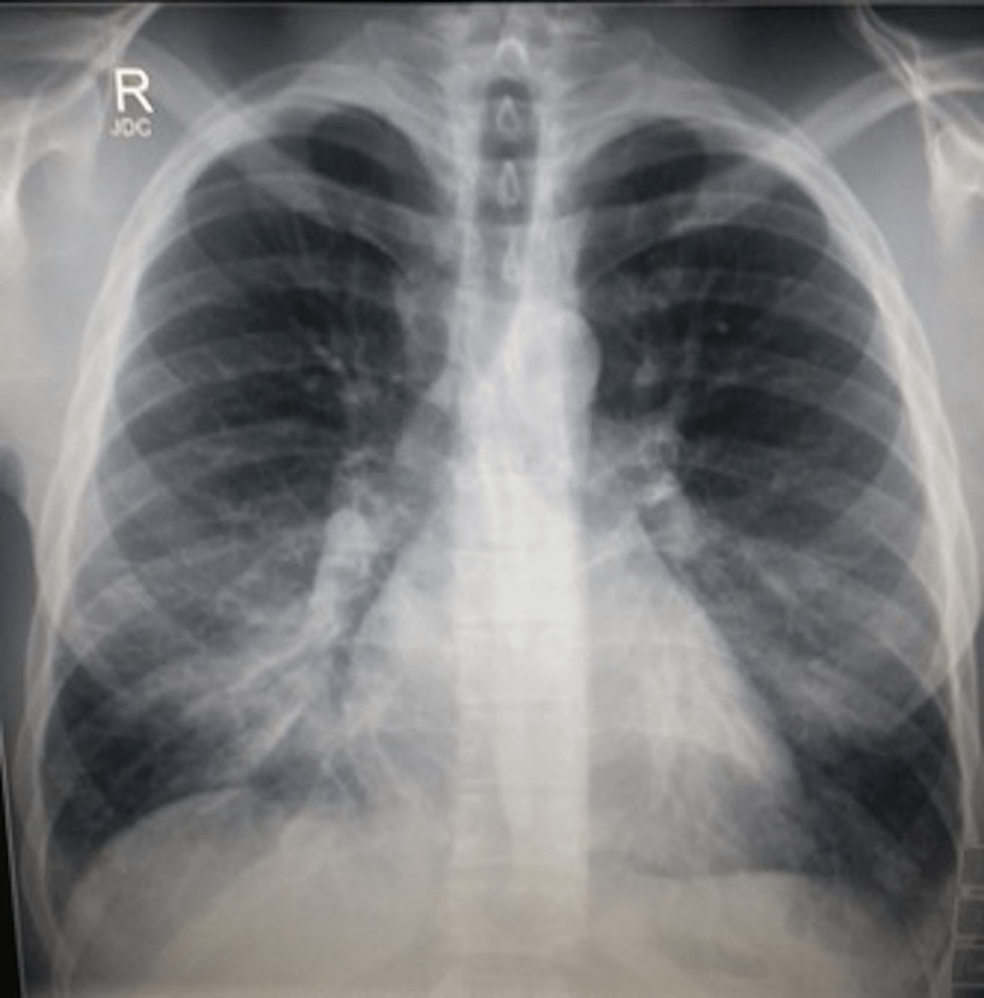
An upright chest radiograph with silhouetting of the right heart border and air bronchograms.
